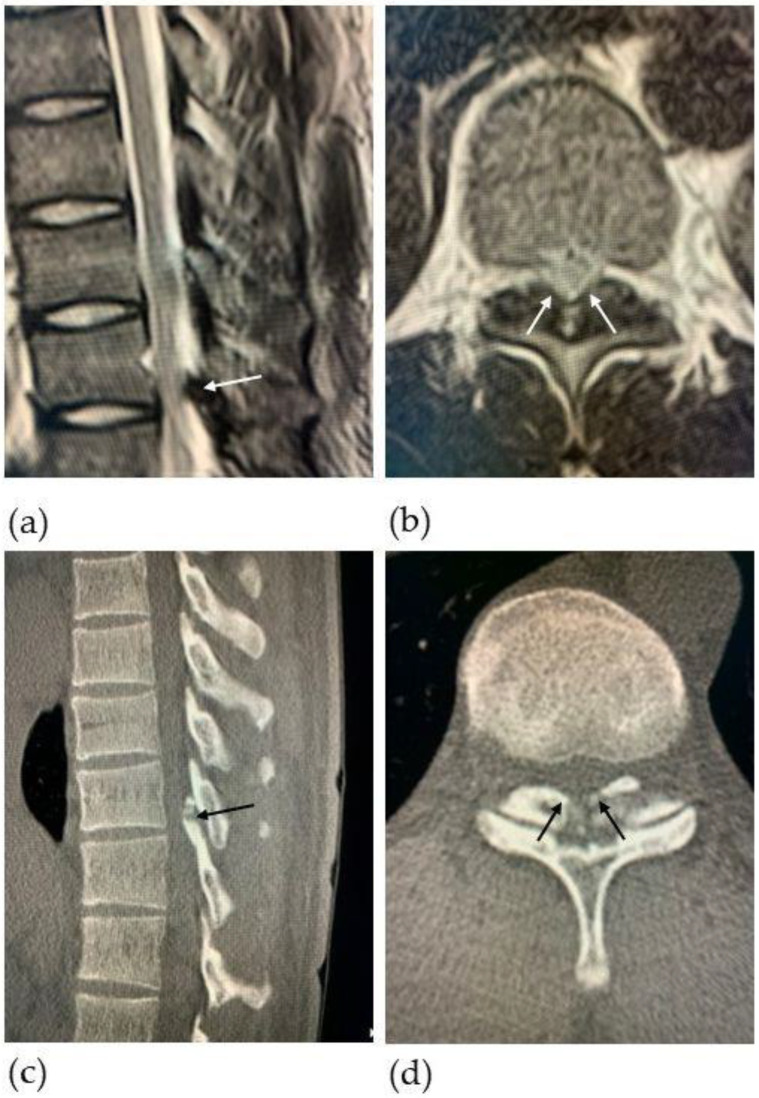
Preoperative magnetic resonance images (MRI) and computed tomography (CT) images. Sagittal (a) and axial (b) T2-weighted MRI of the thoracic spine show spinal cord compression with a high-intensity area at the T10-11 level due to a low-intensity signal lesion (white arrows). Sagittal (c) and axial (d) CT images of the thoracic spine show ossification of the ligamentum flavum (OLF) (black arrows), causing significant canal stenosis.
